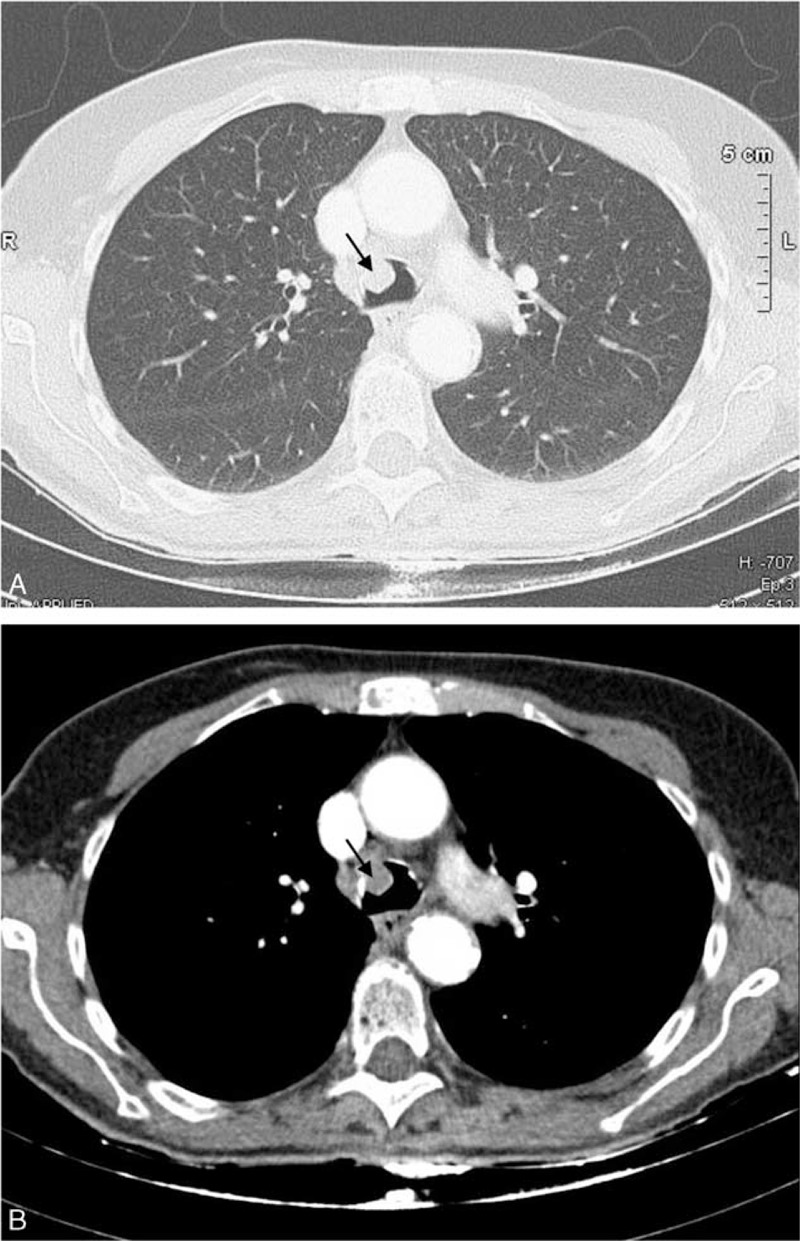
Chest computed tomography (A, Lung window; B, Soft tissue window): endotracheal mass.
